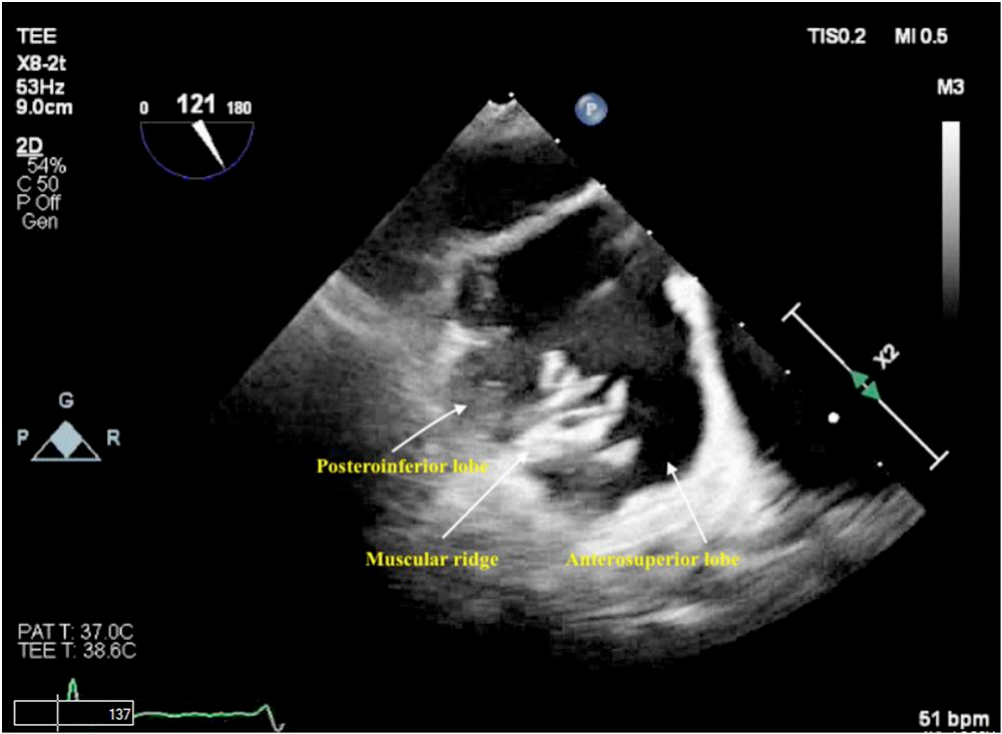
Transoesophageal echocardiogram showing the presence of a bilobed left atrial appendage separated by a muscular ridge.
